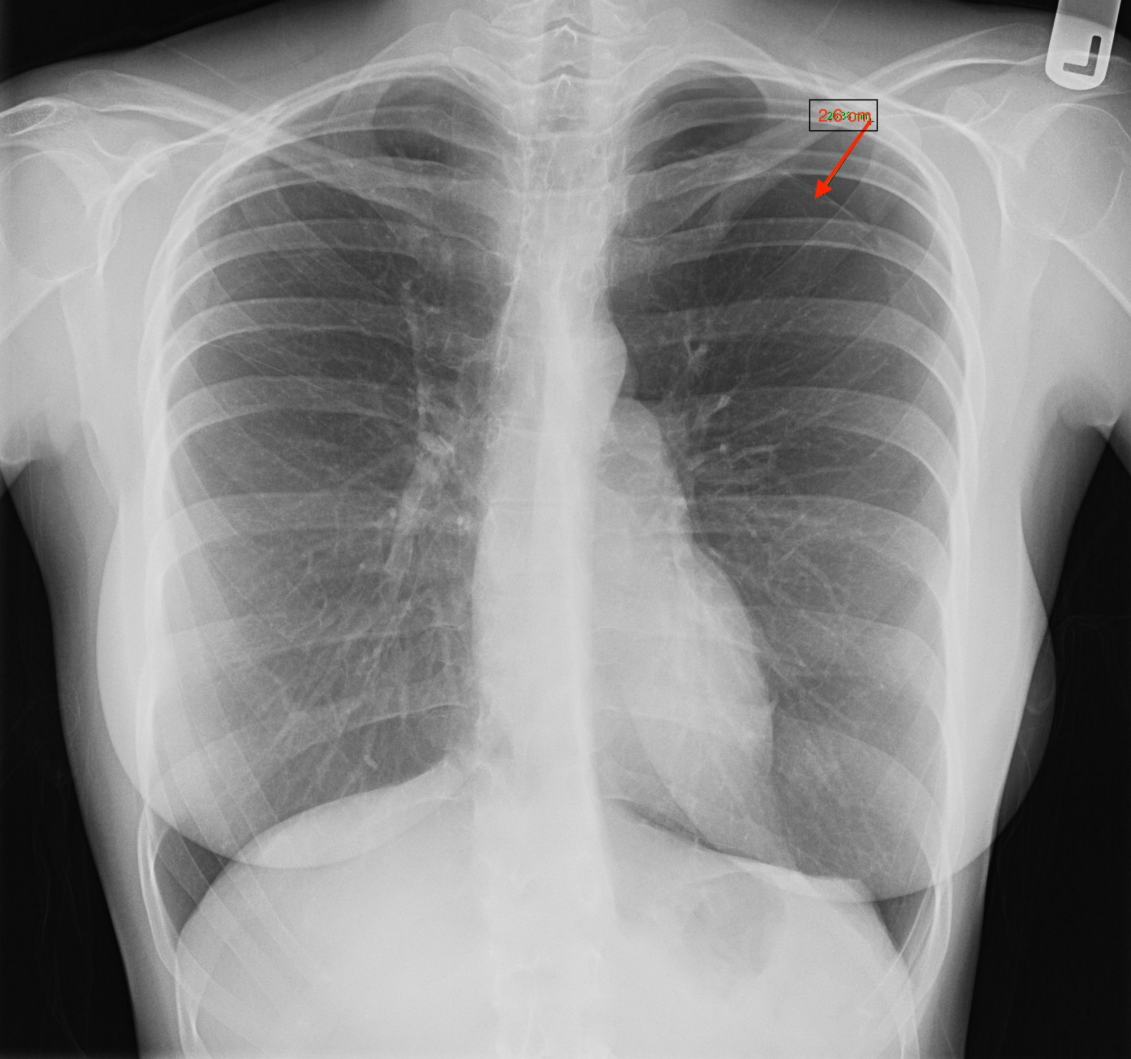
Chest x-ray Chest x-ray (CXR) showing a moderate-sized left pneumothorax with a 2.6 cm gap.
